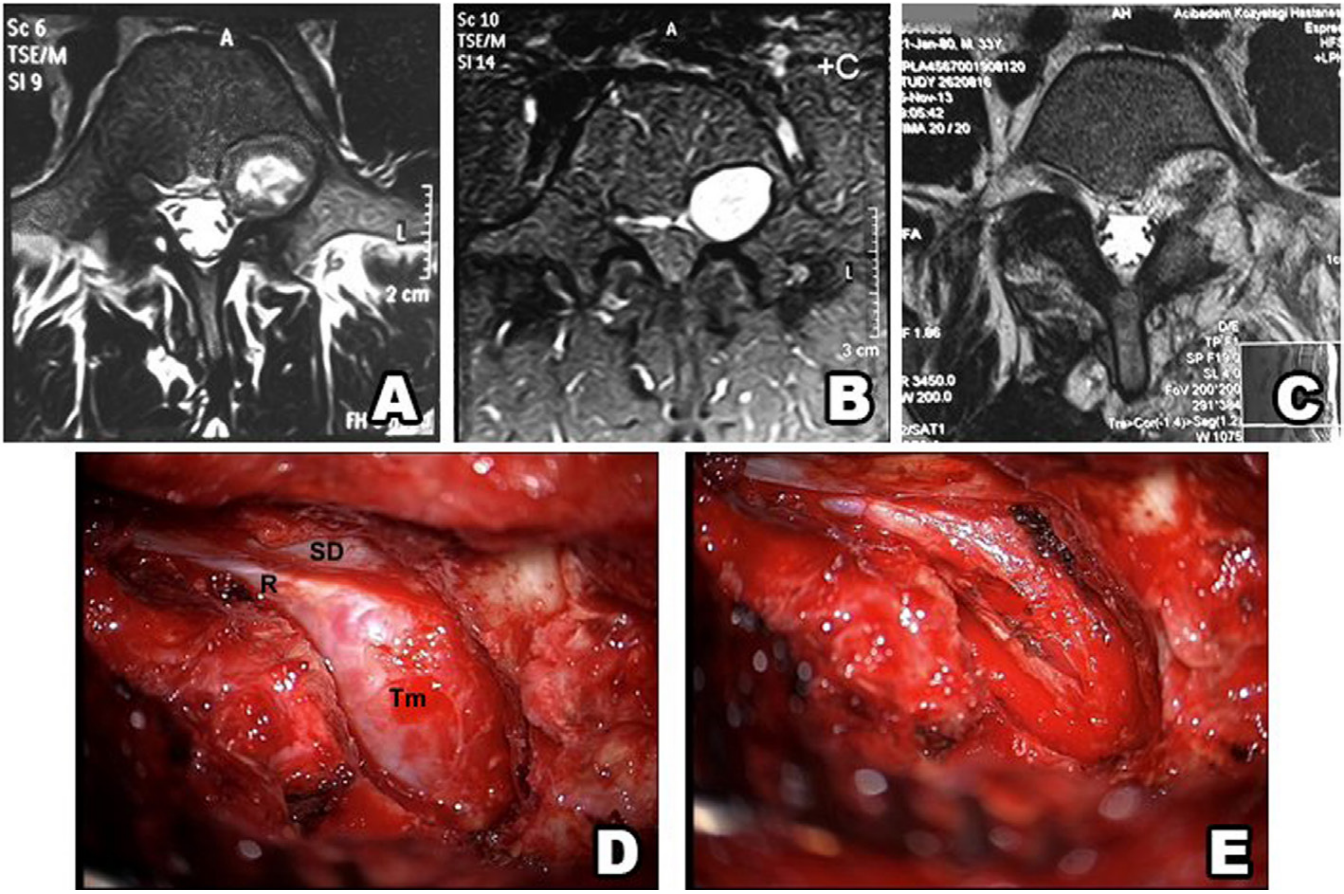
Type IV schwannoma (tumor residing completely outside the root foramina). Preoperative MRI shows a giant cystic spinal schwannoma (A–C). An extraforaminal tumor in the surgical field (D) (SD, spinal dura; R, root; Tm, tumor). Postoperative view of the surgical field shows no tumor residue (E).
